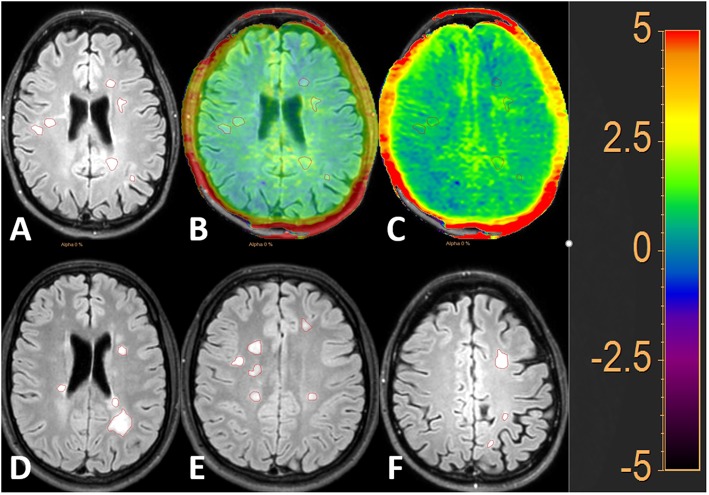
FLAIR image with 50% and 100% APTw image overlay. MS lesions (MSL) are outlined in red. The image to the left shows the lesions on FLAIR (A), the middle image shows the FLAIR image with 50% APTw overlay (B) and the image to the right shows the FLAIR image with 100% APTw overlay (C). Additional examples of the lesion selection are given in (D–F).
